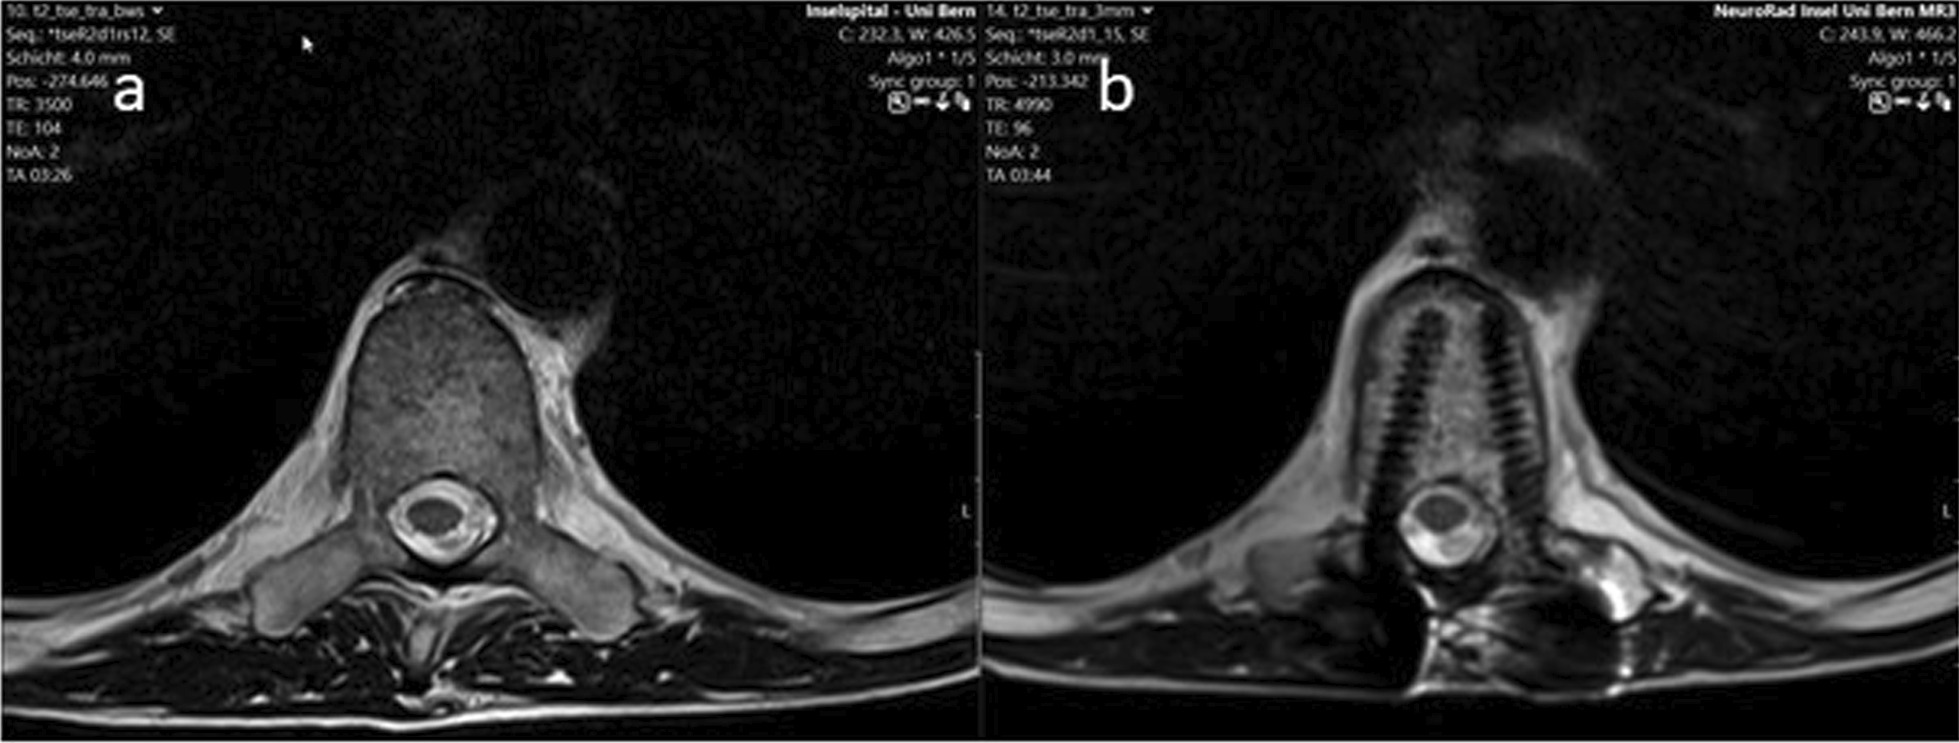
Pre- (a) and postoperative (b) T2 MRI-sequences after stabilization with the CFP-T implants. As shown on b, the spinal cord could be clearly visualized in the proximity of the implants
